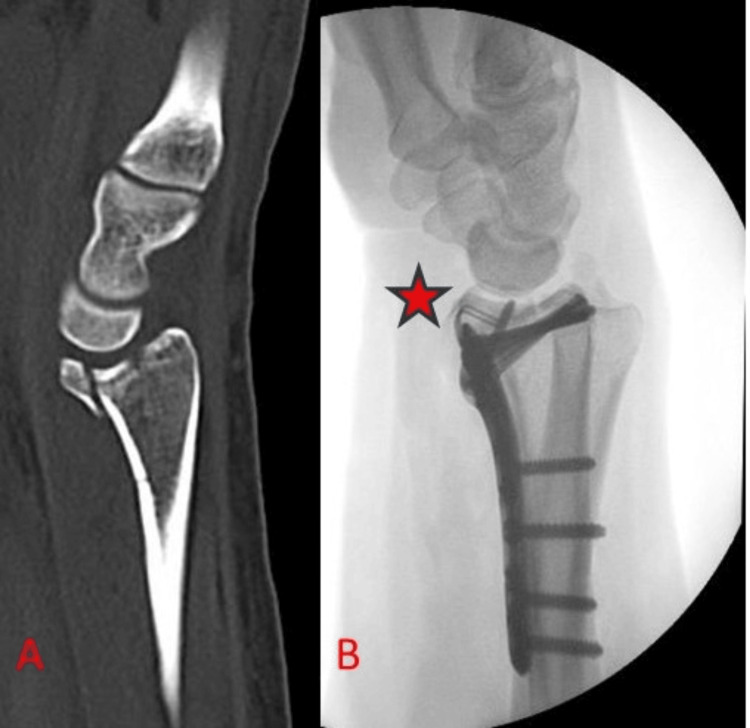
Case example of stabilisation of volar rim fragment (A) Preoperative sagittal CT reconstruction showing a very distal volar rim fragment of the lunate fossa and resulting radiocarpal instability. (B) Intraoperative lateral radiograph showing appropriate placement of hook attachment (noted with red asterisk) to volar locking plate, securing the volar rim fragment.
